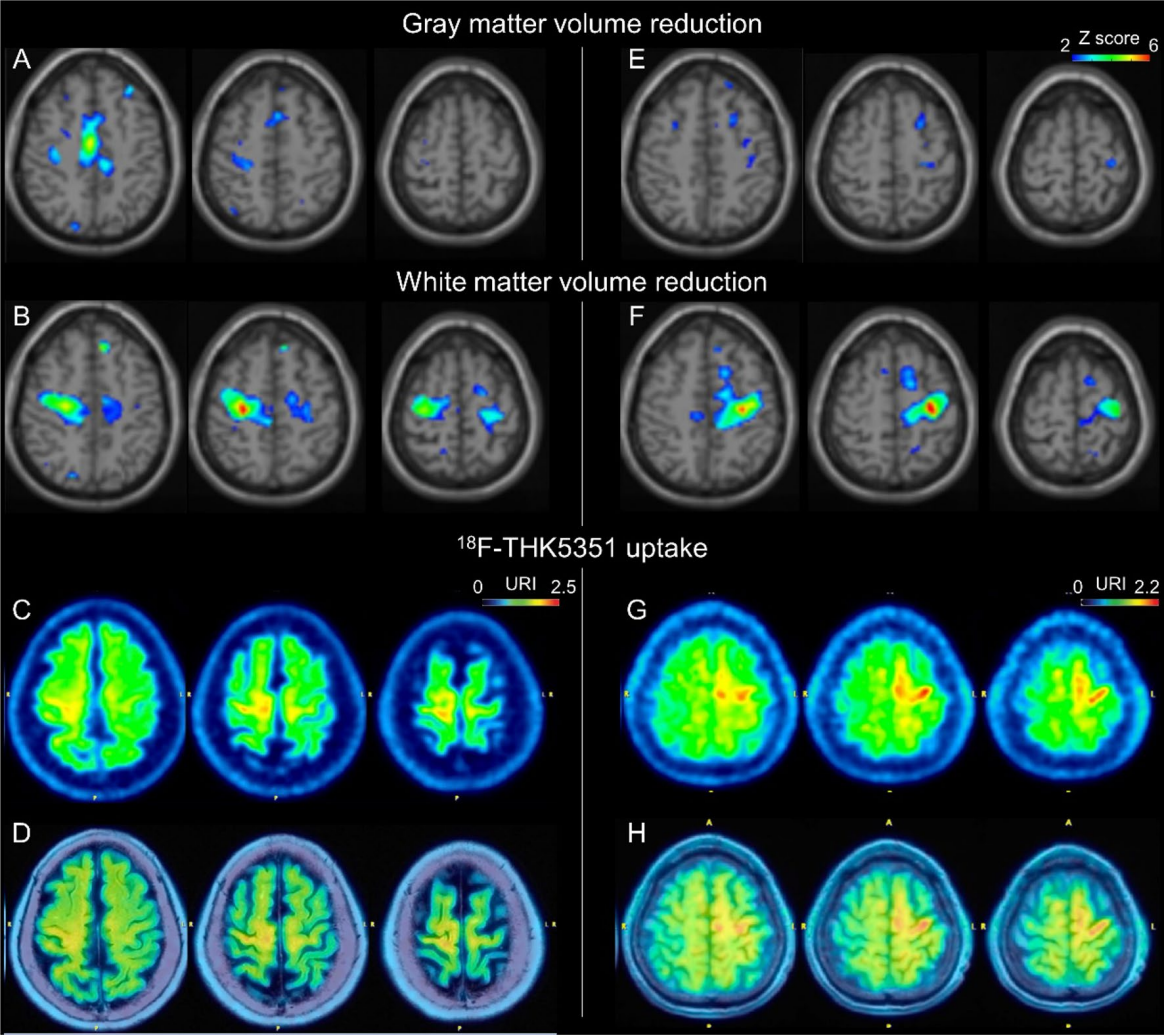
Representative relation between THK5351 uptake and gray or white matter volume reduction analyses. Imaging results for cases 10 (A–D) and 11 (E–H). The results of gray (A,E) and white matter (B,F) volume reduction analyses, ^18^F-THK5351 PET images (C,G), and ^18^F-THK5351 PET images overlaid on T1-weighted MRI (D,H) are shown. *URI* uptake ratio index with the cerebellum as the reference region.
